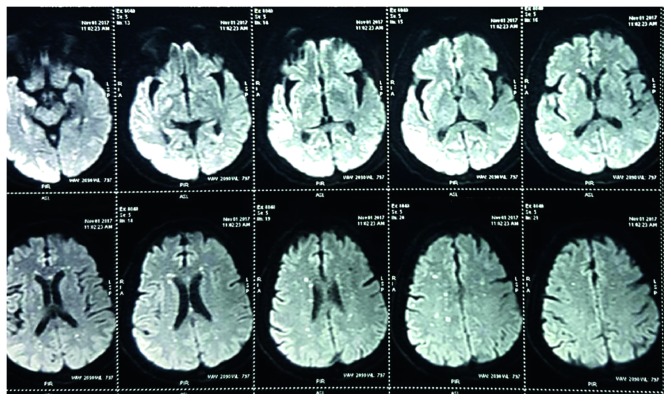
Multiple tiny areas with restricted diffusion in subcortical and periventricular white matter on axial DWI brain.
